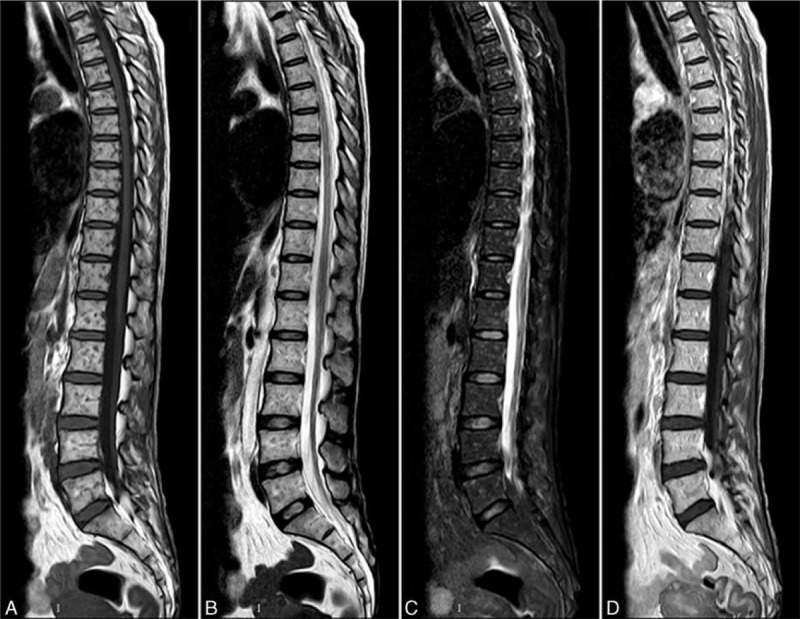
Sagittal spinal magnetic resonance imaging (MRI). Miliary lesions in the vertebral bodies were visualized as a hypo-intense signal on the T1WI (A) and T2WI (B), a hyper-intense signal on the fat-suppression T2WI (C). These signals were enhanced on the T1WI with contrast (D). MRI = magnetic resonance Imaging.
